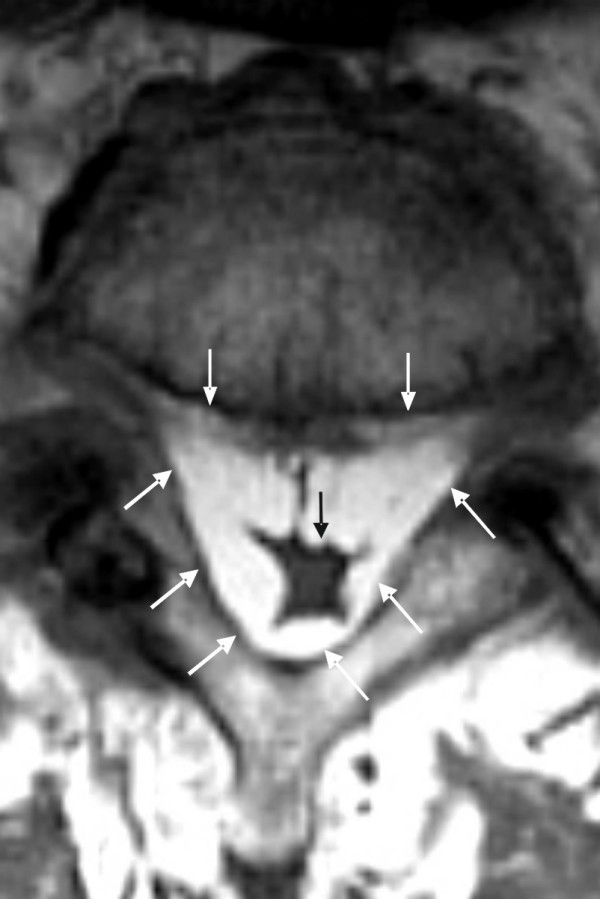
Magnetic resonance images of a true epidural lipomatosis. A T1-weighted cross-sectional image of a true epidural lipomatosis at the lumbar level of LEL 3 (according to Borré). There is a large intraspinal space, which is filled by an excessive amount of epidural fat *(white arrows) *and squeezes the cerebrospinal fluid away so that the dural sac *(black arrow) *completely surrounds the bundle of nerve roots and leaving no free intradural cerebrospinal fluid volume (dural sac cross-sectional area of only 48 mm^2^). These nerve roots are not completely free to move away when a needle is inserted into the dural sac.
